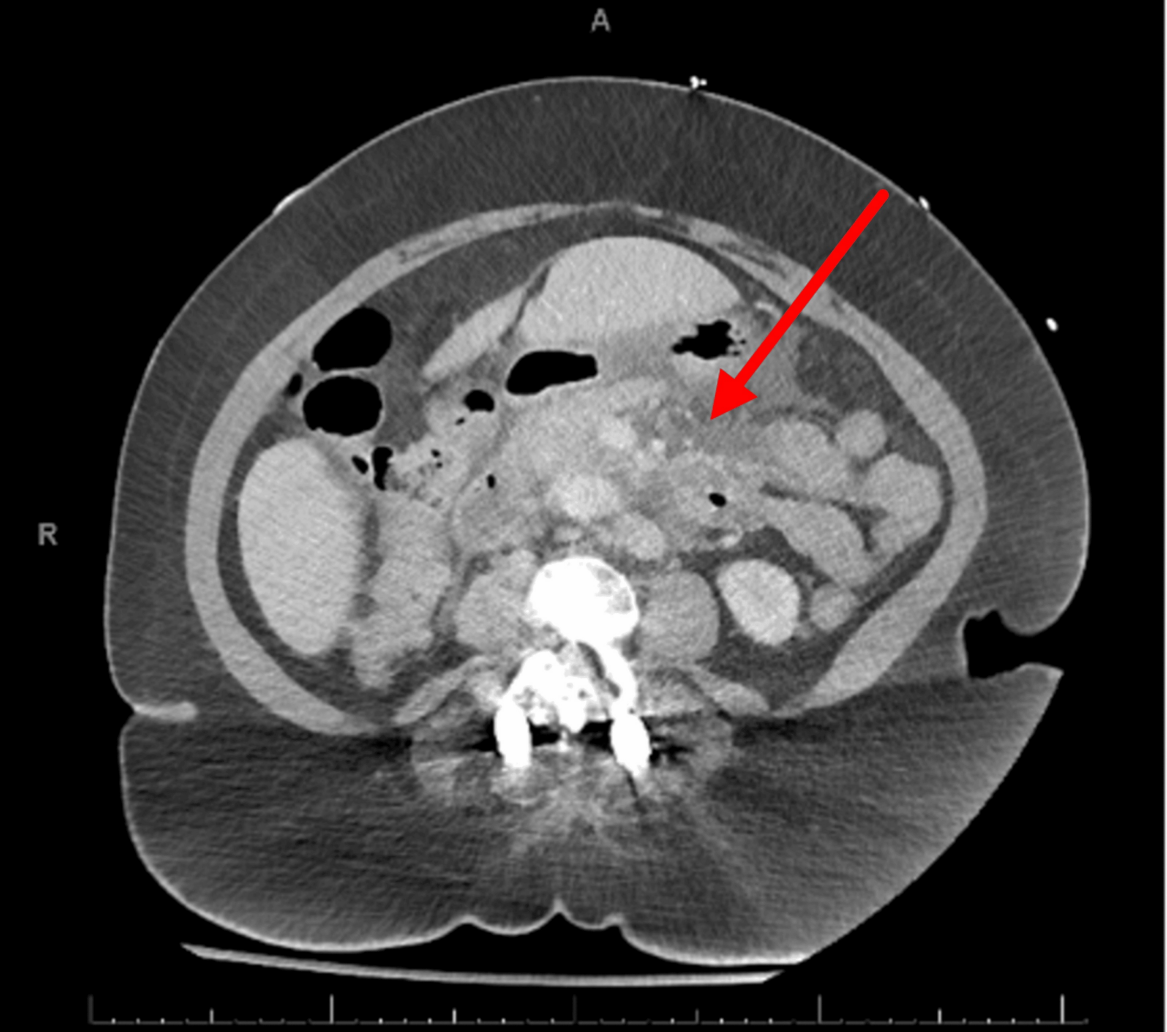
CT of the abdomen CT: computed tomography
